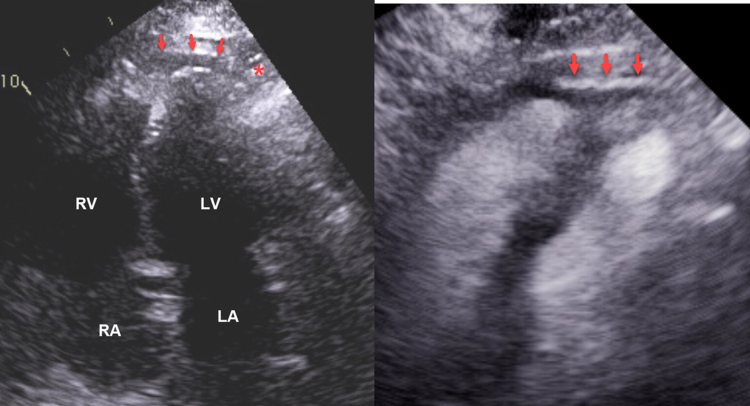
Apical 4-Chamber Views Without (Left) and With (Right) Contrast The images show a thin dissection cavity at the apex (red arrows) that extends to the lateral LV apical epicardium (red asterisk) Contrast and surrounding myocardial contraction (Video 5) indicates that this cavity is not localized effusion or fat. LA = left atrium; LV = left ventricle; RA = right atrium; RV = right ventricle.
